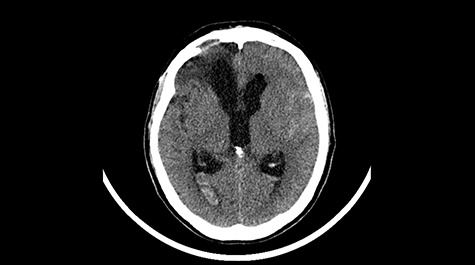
Head CT after polypectomy—presence of blood in fluid spaces, widening of the ventricular system, presence of air in the frontal horns of the lateral ventricles, features of cerebral edema. (Department of Neurosurgery Neurotraumatology and Pediatric Surgery own material).
